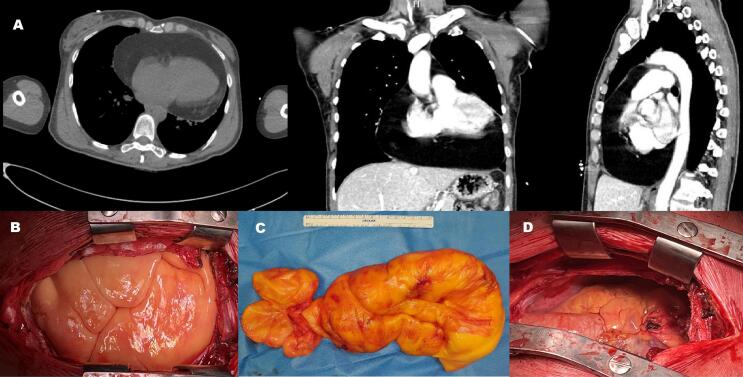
A: Thorax CT scan with sagittal reconstruction; B: view of the lesion after sternotomic incision; C: large lipoid mass extracted from pericardium; D: view of the opened pericardium after mass removal.
